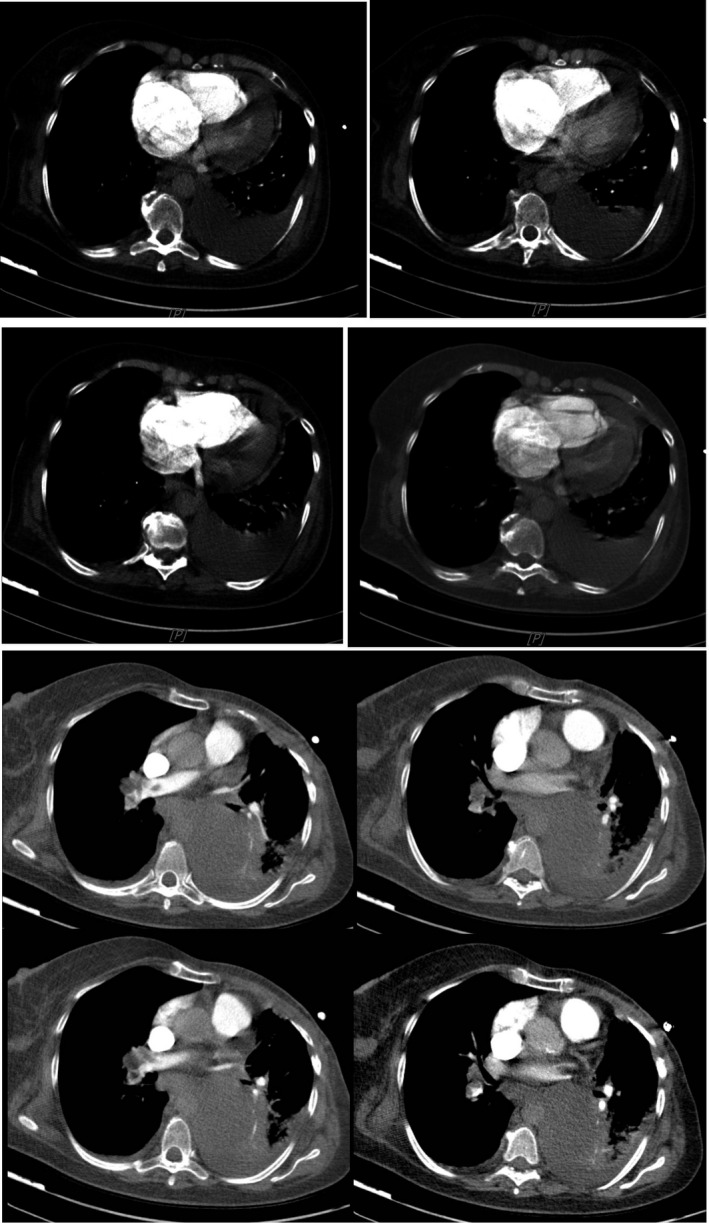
Pulmonary CT angiography demonstrates bilateral pulmonary thromboemboli with RV strain
